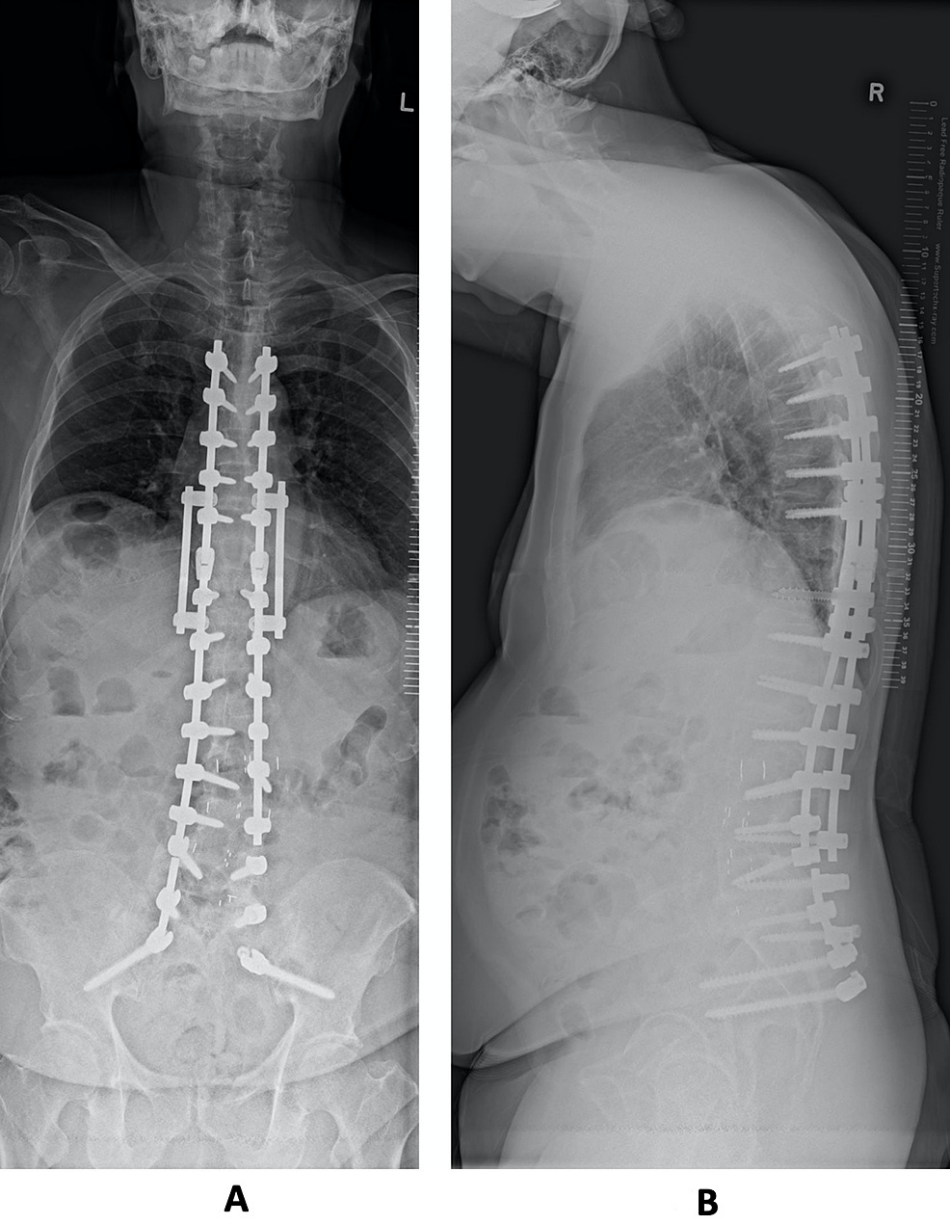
A: AP of the spine demonstrating absence of the broken rod on the left, and a broken rod on the right below the L5 screw. B: Lateral radiograph of the spine showing the absence of the broken rod on one side, with the contralateral rod broken but not migrated. AP, anterior-posterior
